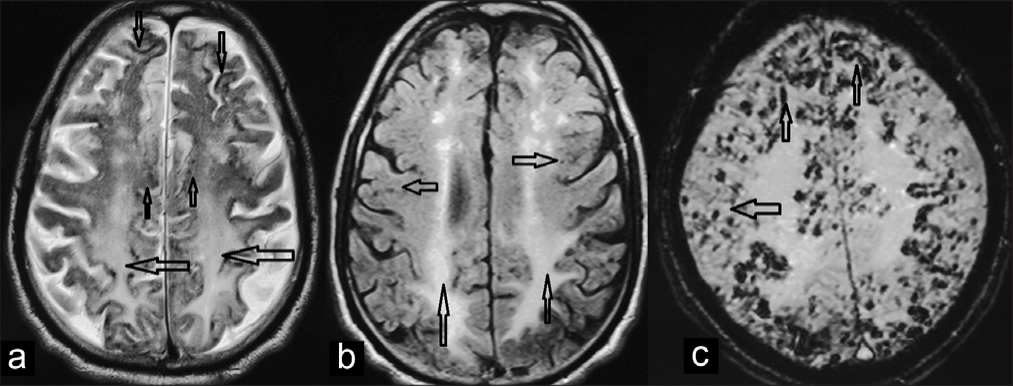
(a) T2 weighted axial MR image showing gyriform hypointense signal intensity foci along the surface of frontal and parietal cerebral convexities of both cerebral hemispheres (downward arrows). Small rounded signal void dots are seen in the cortices (upward arrows) with diffuse hyperintense signal intensity in the white matter (leftward arrows), (b) Axial T2 W fluid attenuated inversion recovery (FLAIR) image through the supraventricular level shows diffuse hyperintense signal intensity in the centrum semiovale bilaterally (upward arrows); small subcortical signal void foci are also seen (right and leftward arrows), (c) Susceptibility weighted (SWI) axial MR image through cerebral convexities above the ventricular level showing prominent gyriform (upward arrows) and innumerable small rounded signal voids (leftward arrow) in bilateral cerebral hemispheres.
